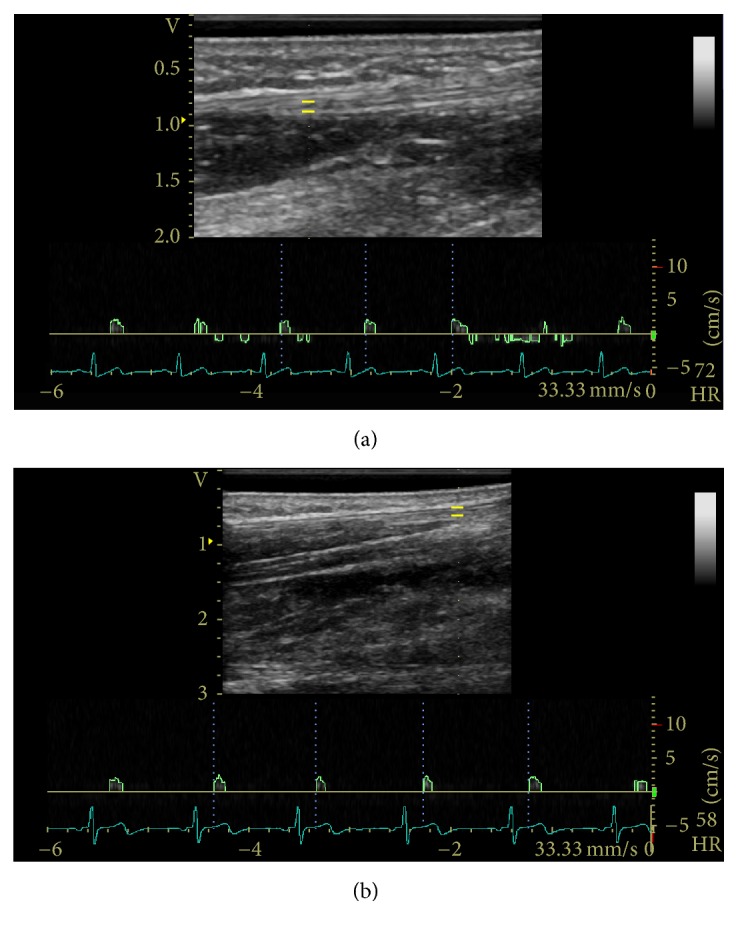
Sample PW Doppler examination in the (a) neutral position and (b) flexed 30° position with spectral tracing of the median nerve proximal to the wrist crease. The yellow bars indicate the gate or sample volume.
